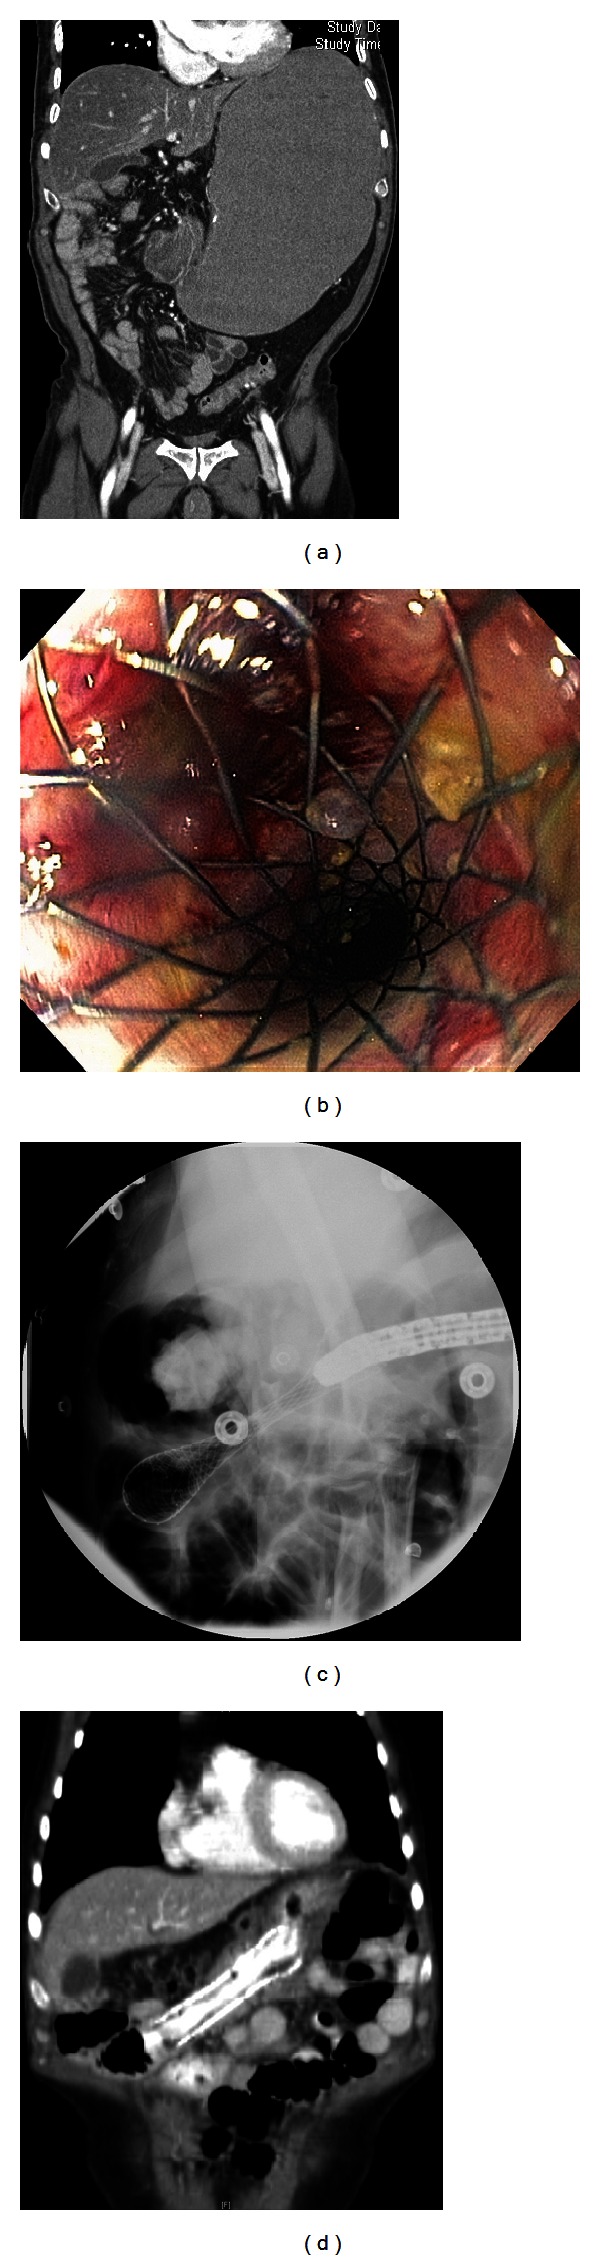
(a) Coronal CT image reveals a malignant gastric outlet obstruction at the gastrojejunal anastomosis. (b) The patient underwent endoscopic placement of a 90 mm × 22 mm WallFlex Enteral Stent across the malignant stricture. (c) Fluoroscopy immediately following stent placement confirms the enteral stent in place. (d) Coronary CT image obtained two months following stent placement confirms the enteral stent in place.
